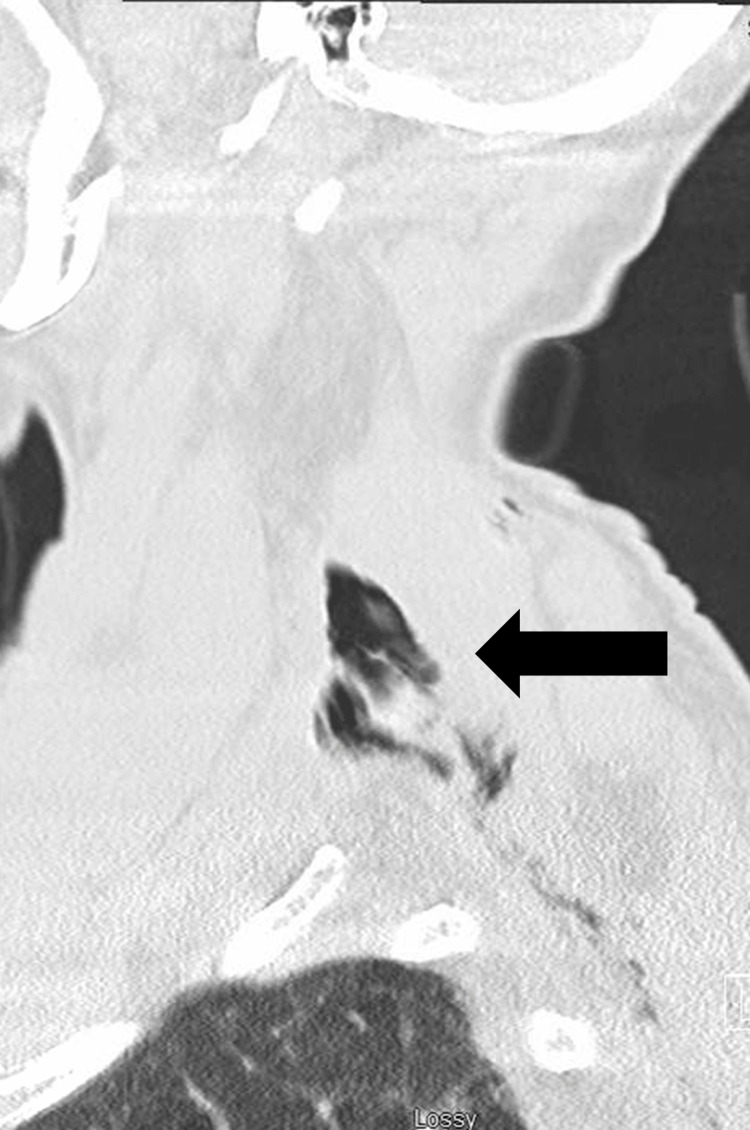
Sagittal view of CT cervical spine reveals subcutaneous emphysema tracking from the posterior thoracic region to the neck, above the apex of the lung. CT: computed tomography
